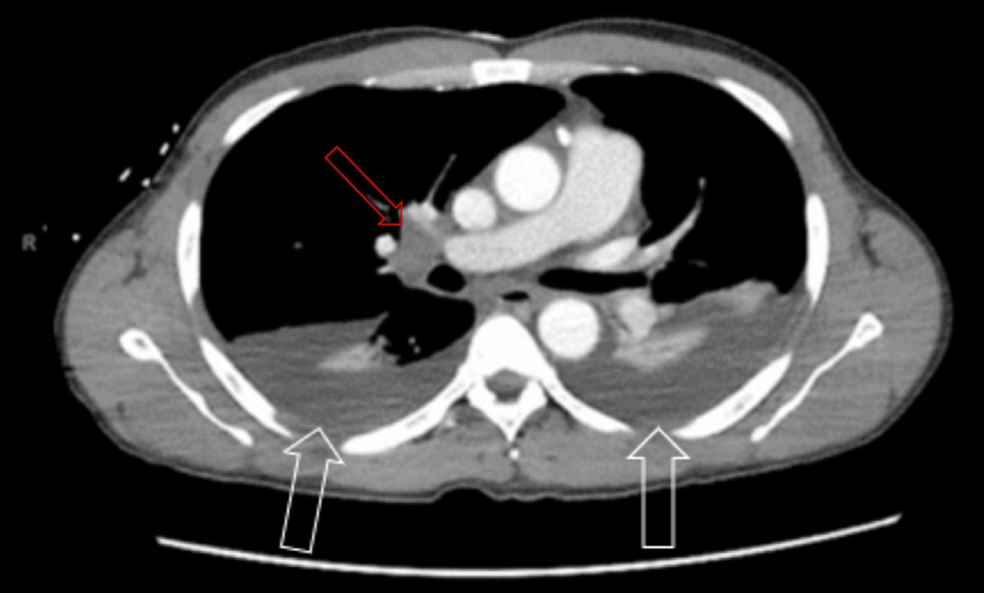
Bilateral pleural effusions (white arrows) with prominent right hilar lymph node (red arrow) visualized on CT
